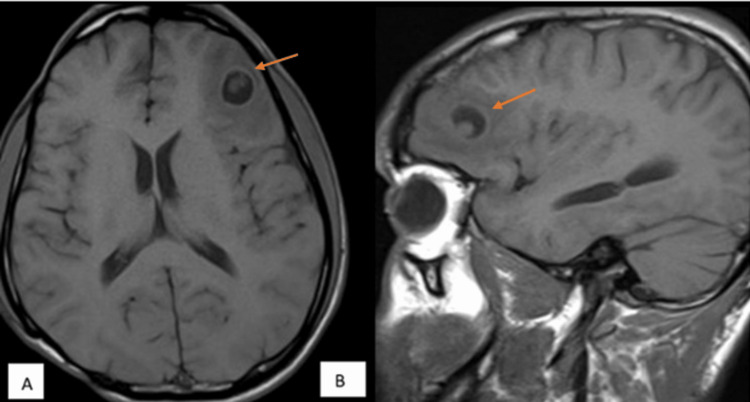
MRI T1-weighted axial and sagittal images showing scolex in a case of neurocysticercosis (A) Axial T1-weighted imaging (T1WI) and (B) sagittal T1WI MRI of the brain (plain) show an oval T1 hypointense lesion in the left frontal lobe (orange arrow in both images A and B) with central T1 hyperintensity suggestive of the scolex.
